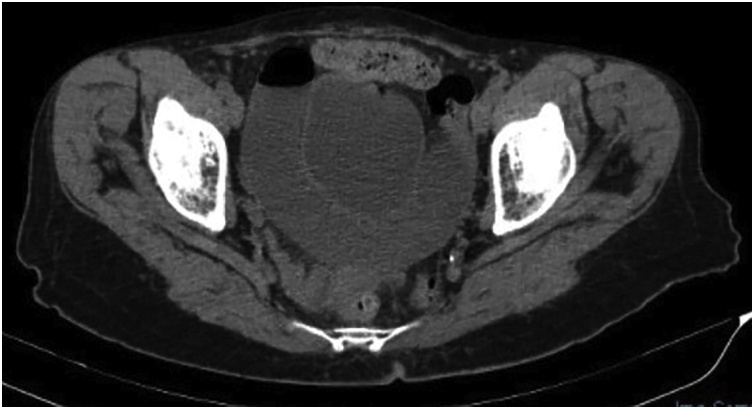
Axial CT imaging showing dilated small bowel loops proximal to the obturator foramen.
